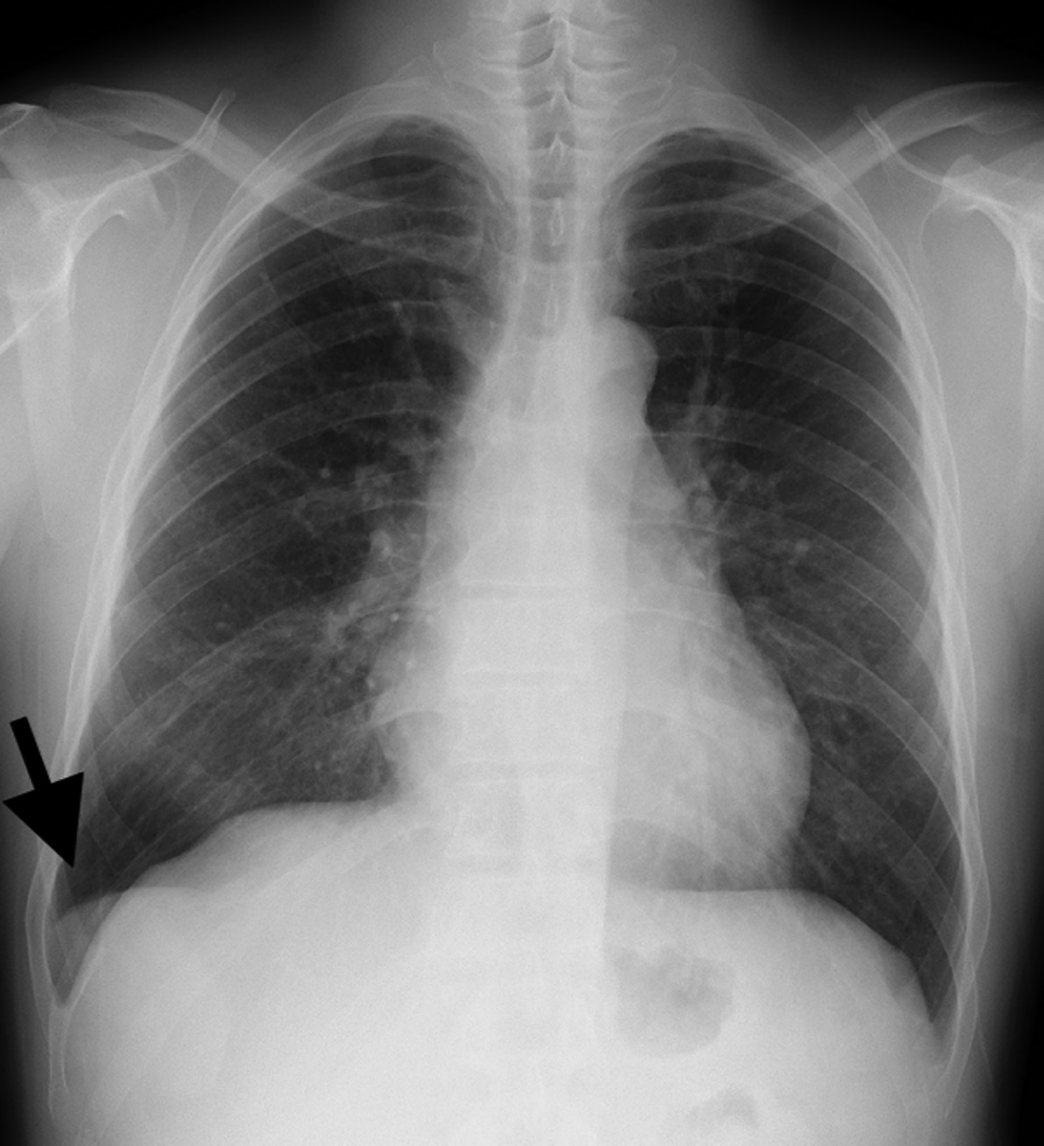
Chest radiograph shows a mass in the lower right thorax (arrow). The border of the right diaphragm is clearly seen
